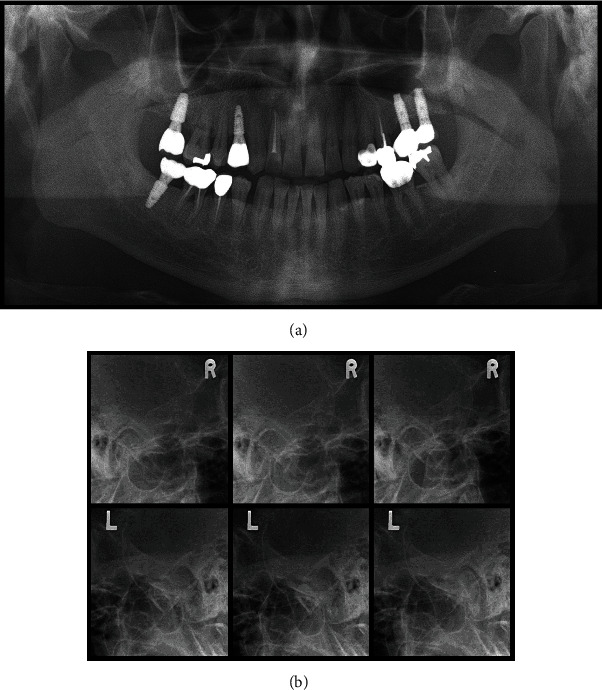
Radiographs of the temporomandibular joints (TMJs). (a) Orthopantomograms of the TMJs showed no significant pathological abnormalities in the temporomandibular joint and jaws. (b) Transcranial view of the TMJs shows no arthritic change in the cortical line of both the joints, and the range of movement of the lower jaw is normal.
